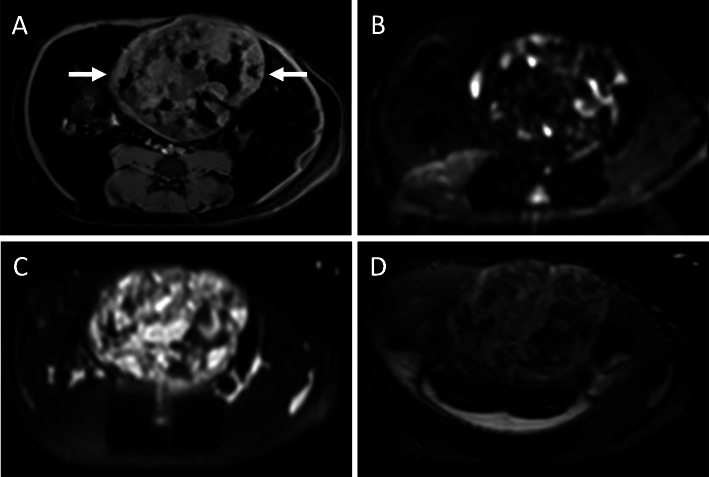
Magnetic Resonance Imaging. a THRIVE image of the teratoma (arrows) with heterogeneously increased signal. Signal voids in the teratoma represent fat on this fat-suppressed imaging sequence. b Diffusion-weighted image with focal areas of increased signal. c T2-weighted TSE image with heterogeneous hyperintensity. d T2-weighted VISTA image with heterogeneous low signal intensity
